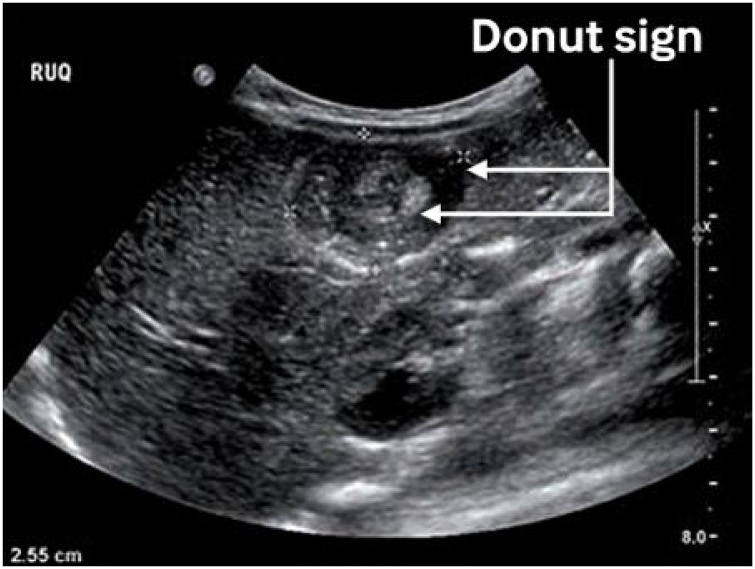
Abdominal ultrasound finding showing characteristic donut appearance.
